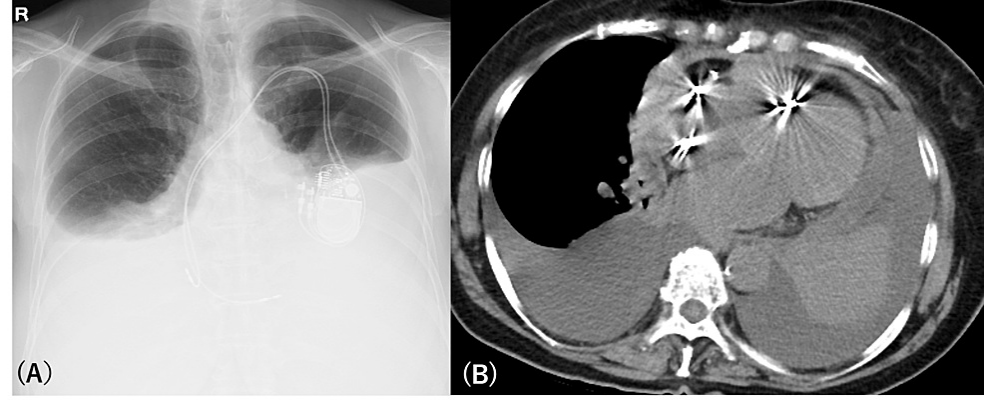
(A) Chest X-rays at the time of transfer to our hospital. (B) The axial view of CT of the chest showed small pericardial effusions and progressive bilateral pleural effusions at the time of transfer to our hospital
